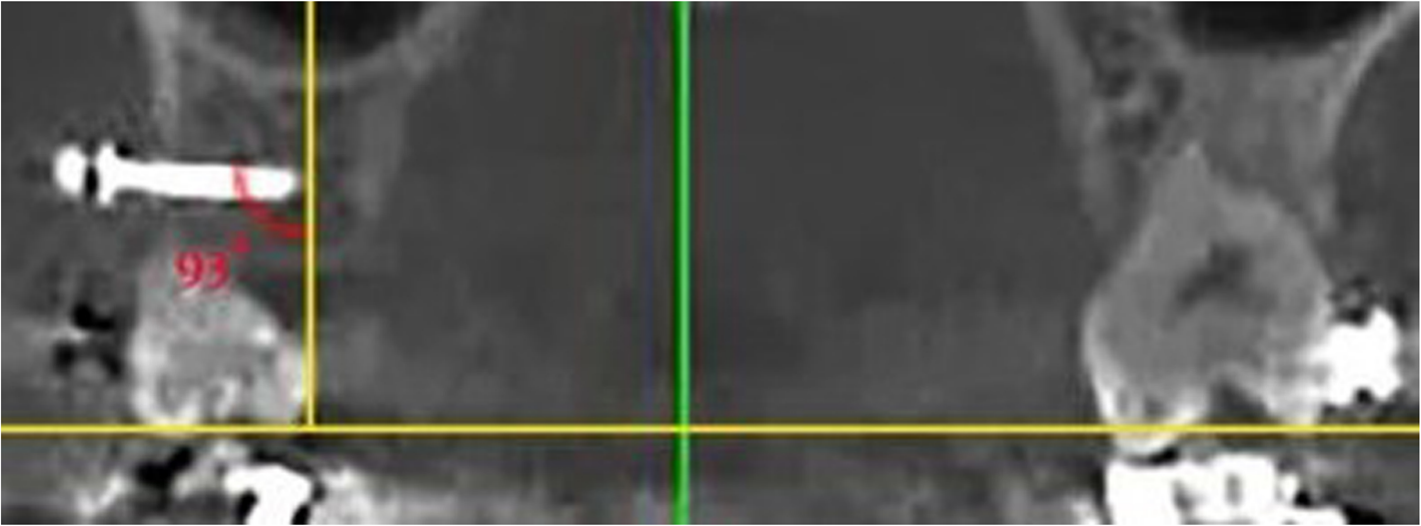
Coronal cross-sectional CBCT image for measuring the insertion angle of miniscrews
